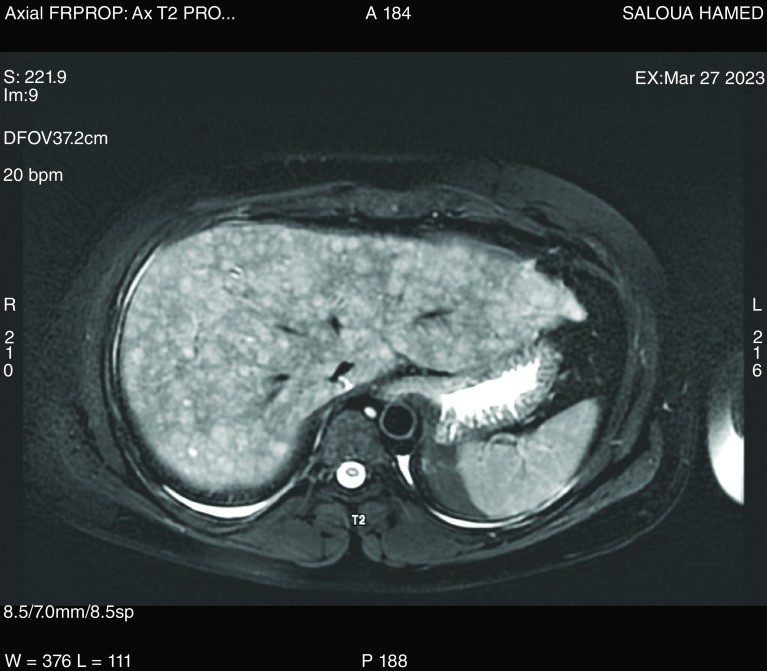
Liver MRI revealing a multiple nodular infiltration of the liver, with hyperintense signals on T2-weighted imaging.
